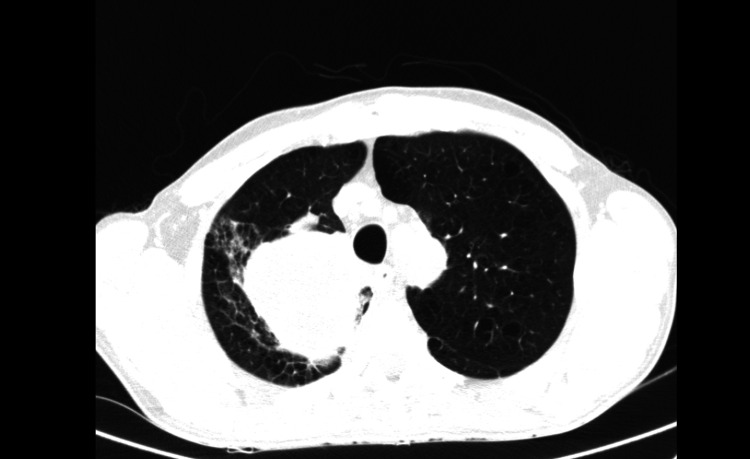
Follow-up abdominal and chest CT after two cycles of chemotherapy in August 2020
